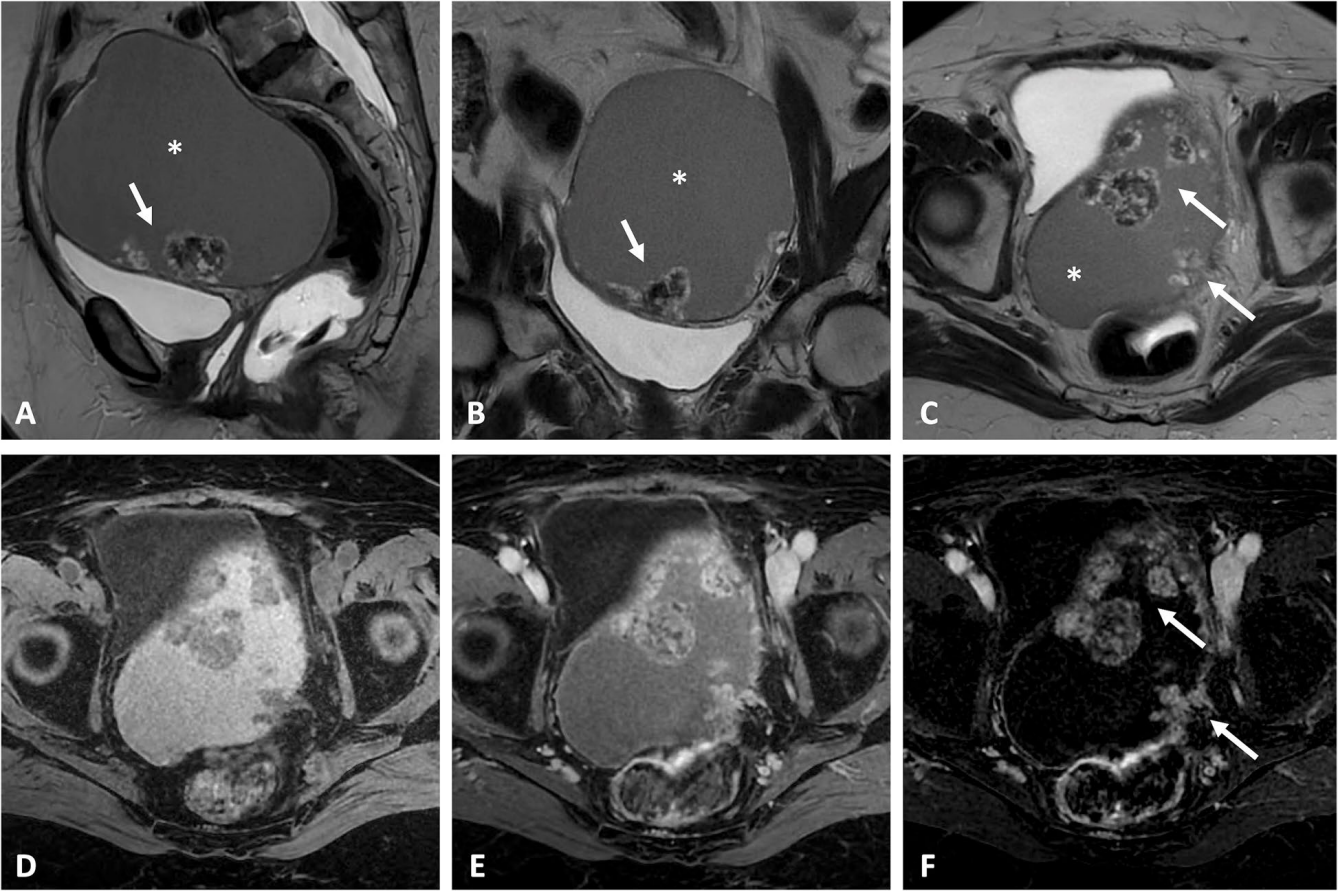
Borderline seromucinous tumor within an ovarian endometrioma in a 77-year-old woman with a long-standing history of endometriosis and previous hysterectomy for cervical cancer. Sagittal (a), coronal (b), and axial (c) T2-weighted images show a mixed ovarian mass filled with typical intermediate signal intensity content (*“shading” effect), containing multiple mural solid nodules (arrows). Axial fat-suppressed T1-weighted image (d) shows the homogeneous hemorrhagic fluid classically found within ovarian endometrioma. Heterogeneous enhancement of the nodules is displayed on fat-suppressed contrast-enhanced T1 image (e), better seen on subtraction sequence (f)
